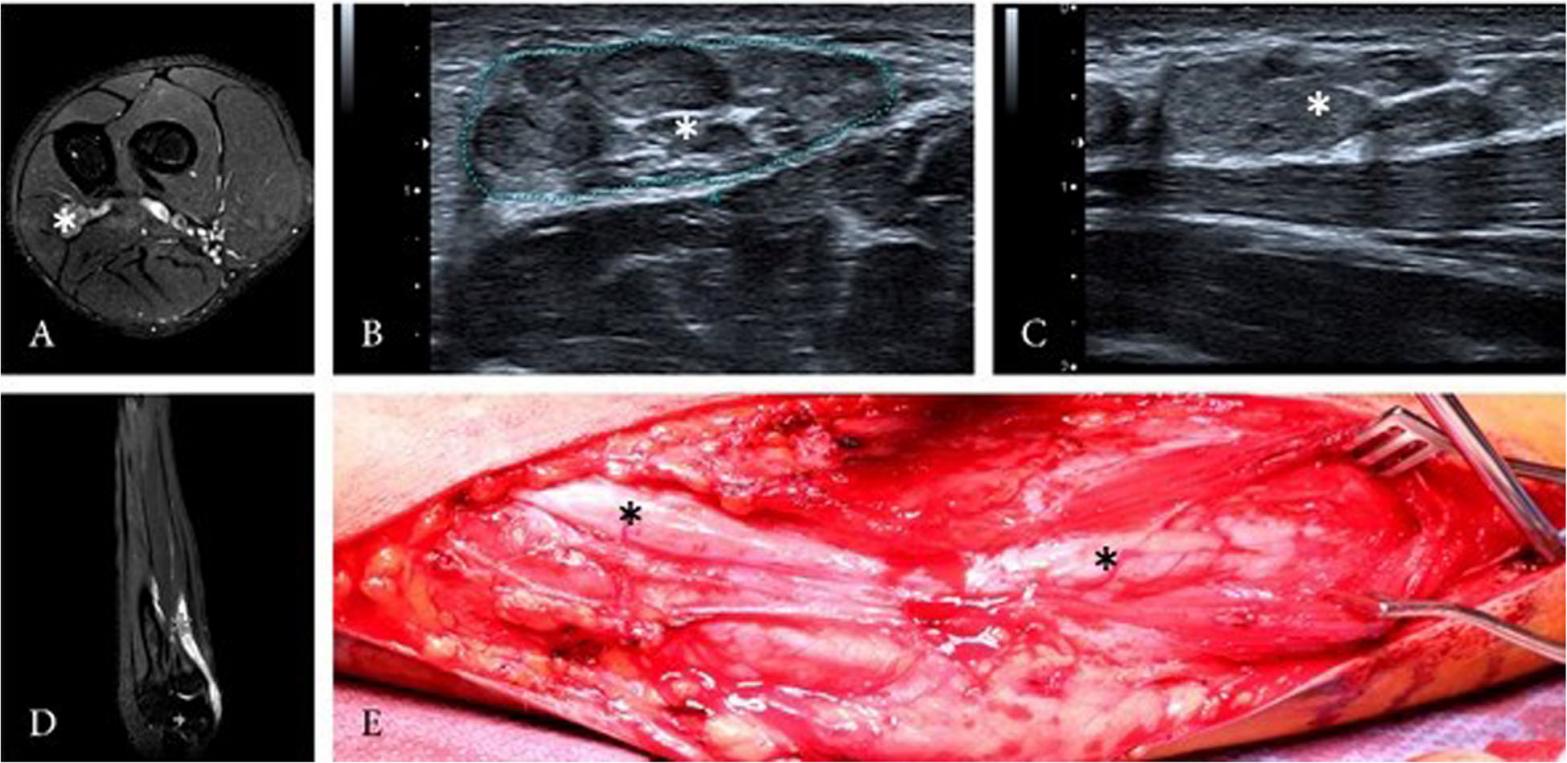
HRNS (b + c) and MRI (a + d) of the left forearm depicted nerve enlargement (asterisk) with perineurial tissue and still identifiable fascicular structure. Intraoperative view showing the perineurioma (e)
